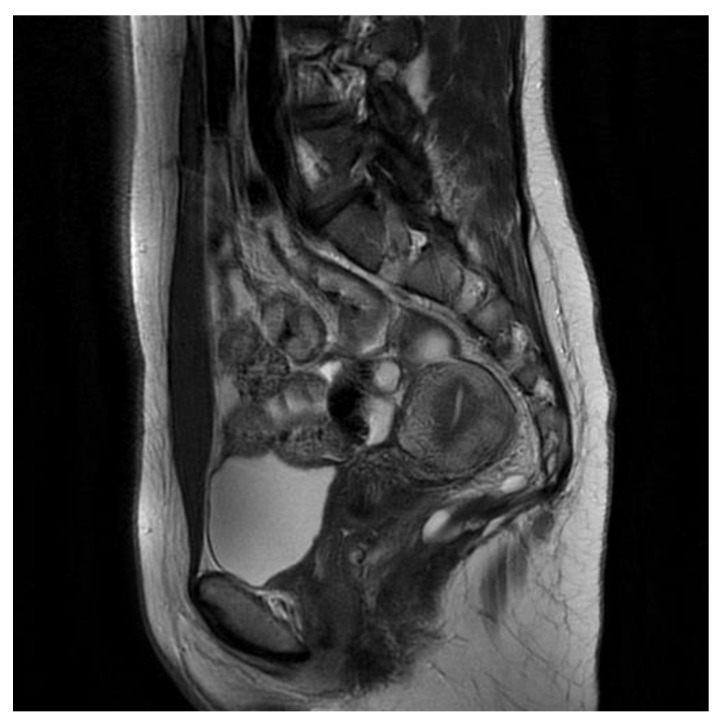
Second patient MRI scan of the lesser pelvis eight months after the procedure.
